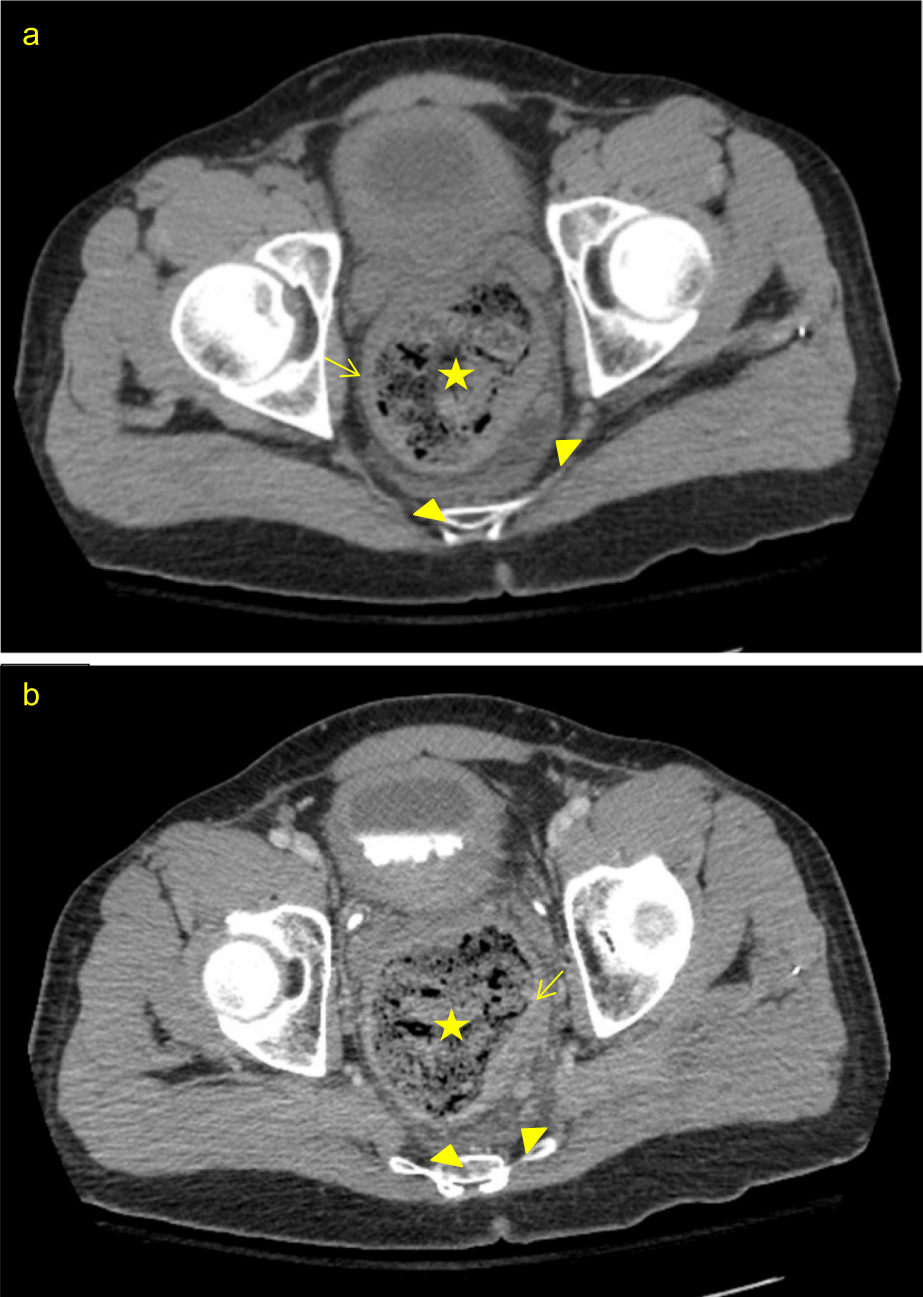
Axial pre-contrast (a) and contrast-enhanced (b) CT images of a 30-year-old male with stercoral colitis demonstrate rectal dilatation (star) due to fecal impaction. Rectal wall thickening (arrow) and perirectal fat stranding (arrowheads) are also seen as a result of inflammation
